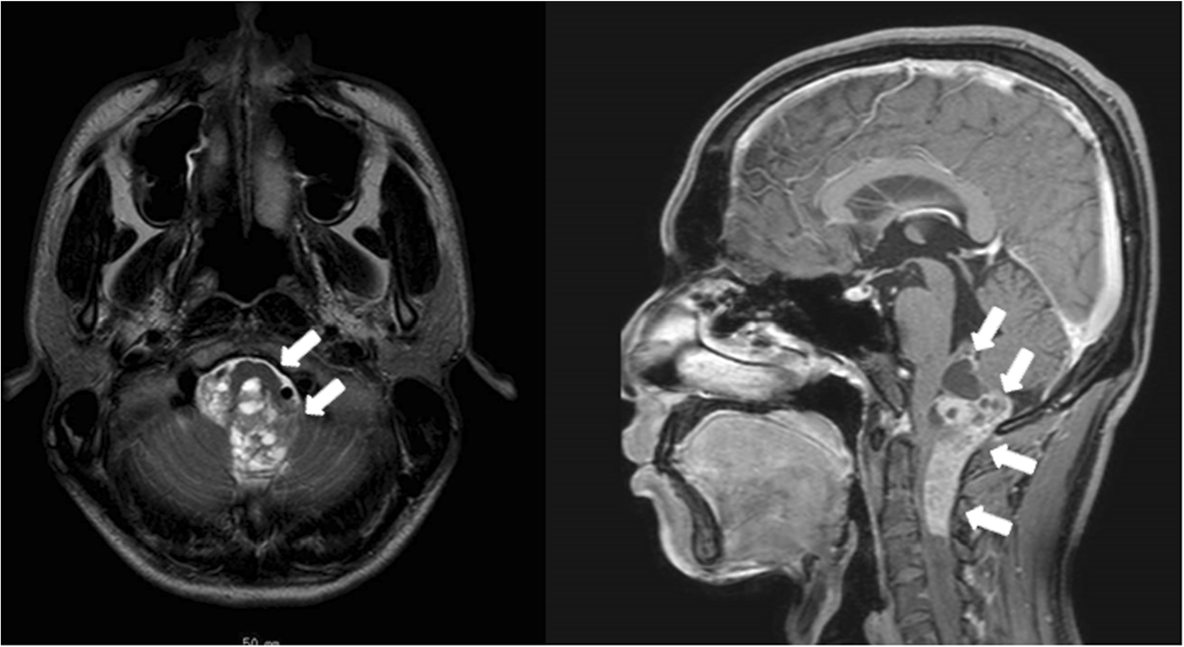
Head MRI. The MRI scan reveals an anaplastic ependymoma that extended from brainstem to forth ventricle
